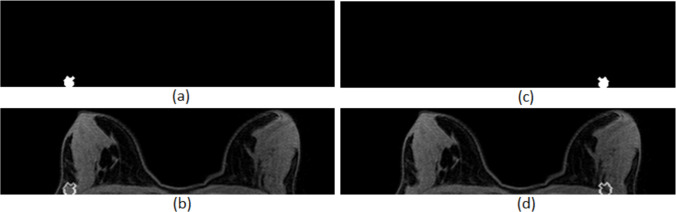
Control ROI creation: a extracted ROI, b outline of the extracted ROI overlaid on the MRI slice, c created control ROI, and d outline of the control ROI superimposed on the MRI slice
